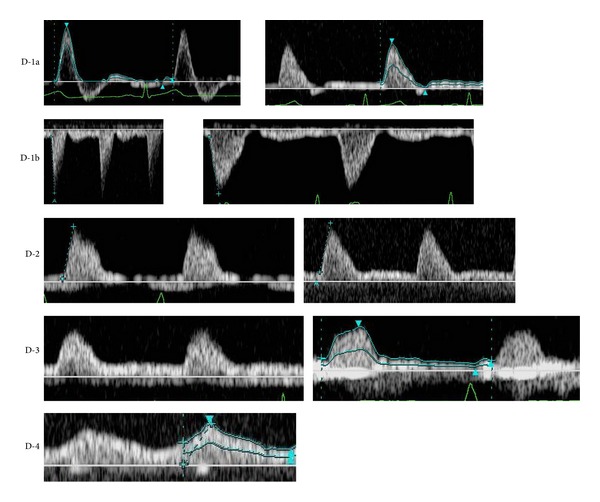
Novel classification of the vascular waveform of flap-feeding vessels in Doppler ultrasonography. D-1a type: normal waveforms in which systolic crests rise steeply, followed by reflux components; D-1b type: normal waveforms in which systolic crests rise steeply but reflux components are lost, and a notch is noted between systolic and diastolic waves; D-2 type: peaks are formed, but the width of systolic crests is wider than normal, reflux components are lost, and no notch is present between systolic and diastolic waves; D-3 type: systolic crests are moderate and form no peak; and D-4 type: moderate continuous waveforms.
